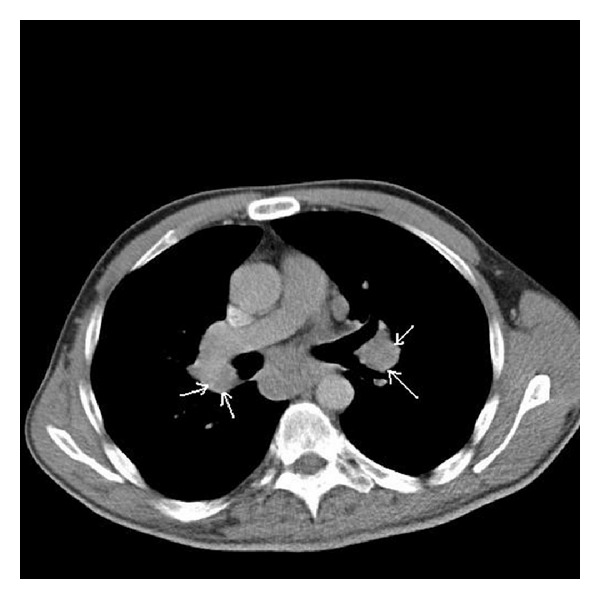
Thorax CT showed multiple mediastinal and hilar lymphadenopathies.
